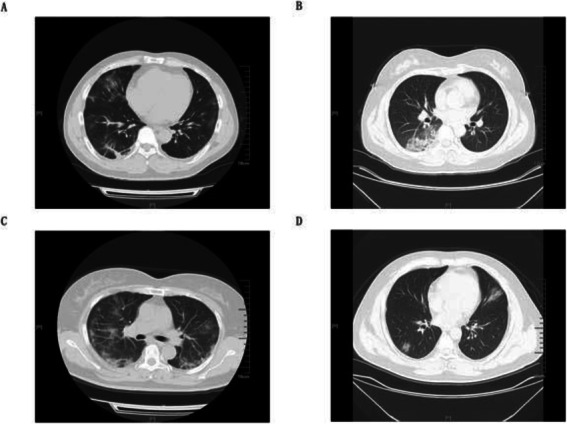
Full-body CT scan showing an extended subcutaneous emphysema, pneumomediastinum and pneumopericardium
